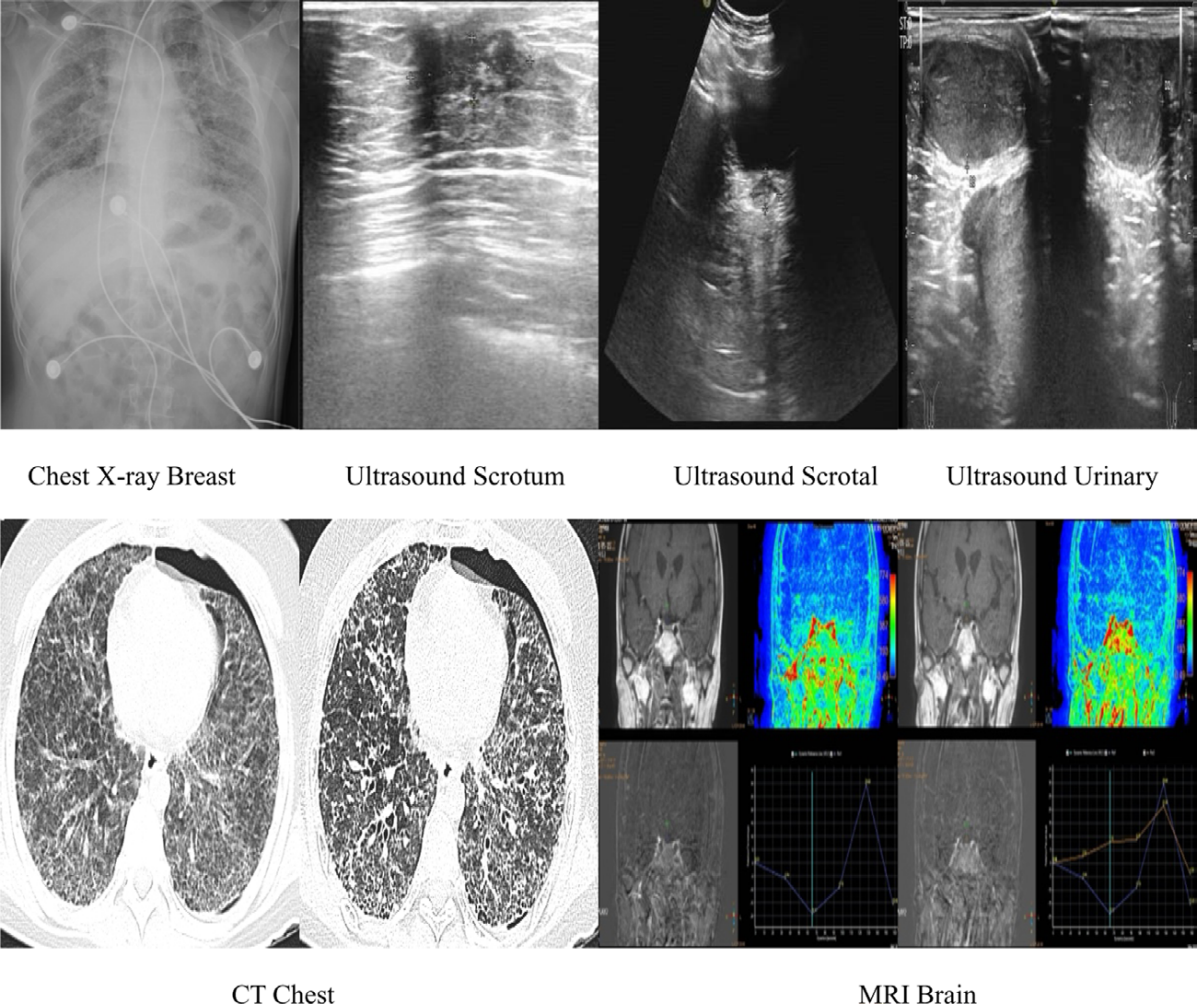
Ultrasound scrotum, ultrasound scrotal, ultrasound urinary, chest X-ray breast, CT chest, and MRI brain. CT = computed tomography, MRI = magnetic resonance imaging.
